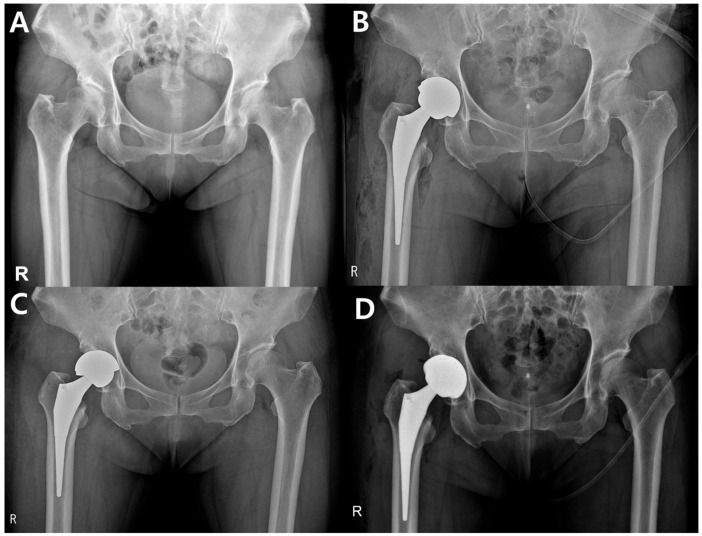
Early acetabular cup fixation failure case. (A) Preoperative radiograph of a 61-year-old female with right hip osteoarthritis. (B) Primary total hip arthroplasty using a ceramic monoblock acetabular cup (Maxera). (C) Early acetabular cup fixation failure observed on postoperative day 7. (D) Revision total hip arthroplasty using an acetabular component with a modular ceramic liner. The letter “R” in the lower left corner indicates the right side.
